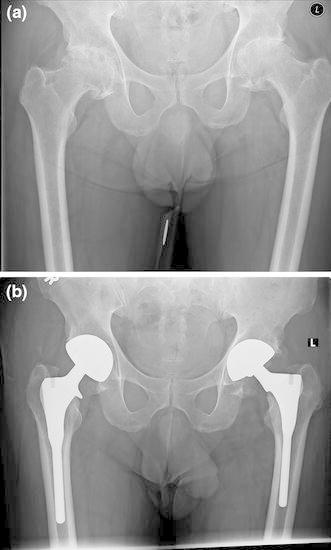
a, b Pre- and post operative radiographs of staged bilateral dual-mobility socket primary THA in a 73-year-old male with severe Parkinson’s disease
